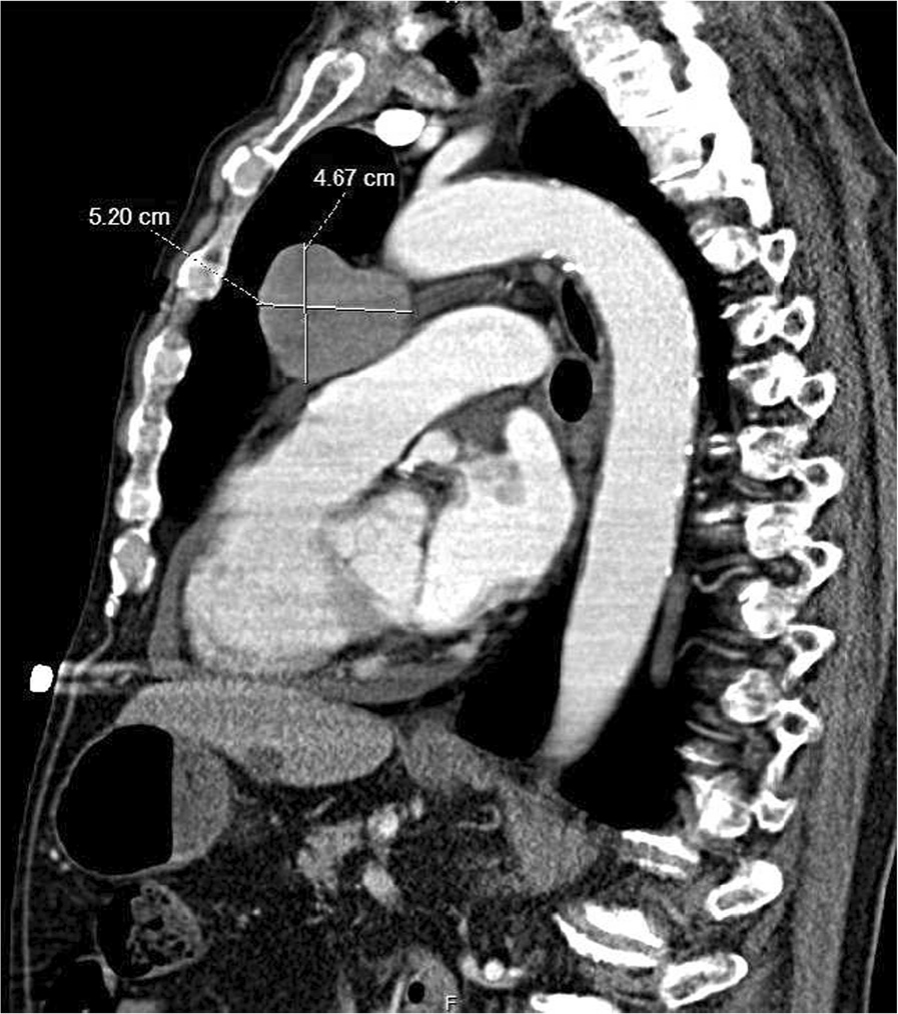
CT scan of the chest. Sagittal CT scan image of the chest reveals a large mediastinal mass measuring 5.2 × 4.7 cm. There is no hilar or mediastinal lymphadenopathy.
